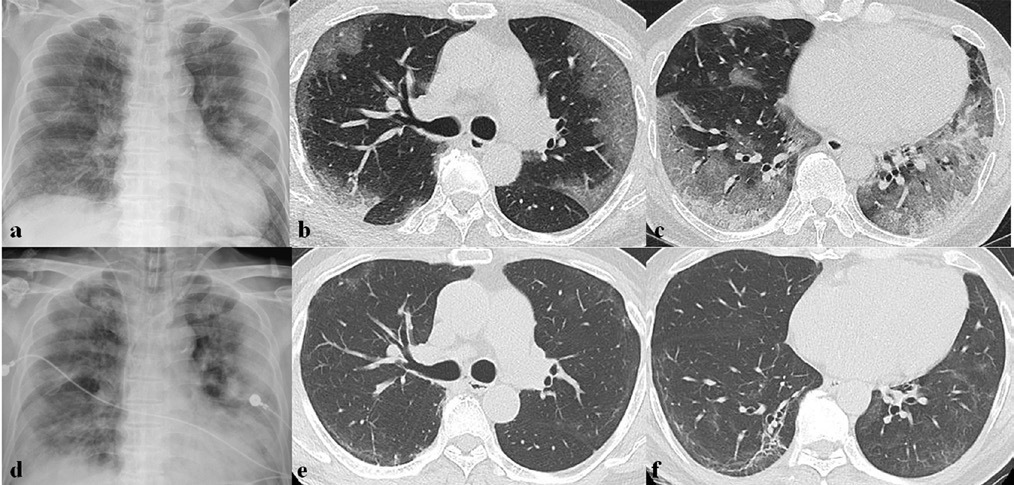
A 64-year-old male patient with SARS-CoV-2 infection. (a) Initial chest radiograph (CXR) obtained 7 days after symptom onset shows bilateral multifocal patchy ground-glass opacities (GGOs) with peripheral predominance. (b, c) Chest CT scans obtained on the same day with (a) show multifocal patchy and diffuse GGOs with the crazy paving appearance and mixed GGO and consolidation in both lungs. (d) Follow-up CXR after 2 days of (a) shows rapid progression with bilateral diffuse consolidations and GGOs. (e, f) Follow-up chest CT scans obtained 1 month later show markedly decreased extent of GGO with remnant subpleural line and traction bronchiectasis, suggesting pulmonary fibrosis.
